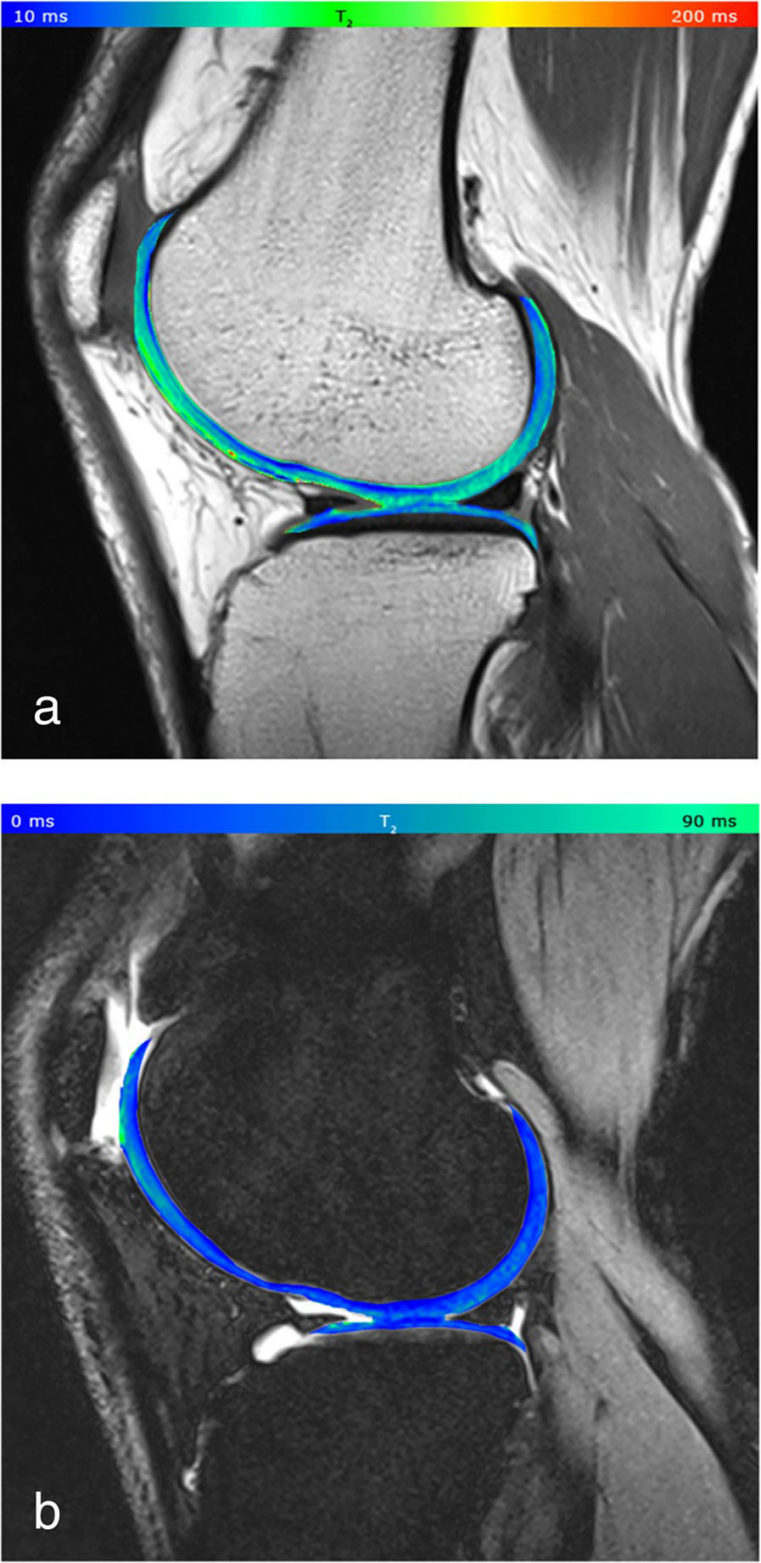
a Cartilage T2 map acquired with a Carr-Purcell-Meiboom-Gill (CPMG) multi-echo spin-echo (MESE) sequence, pseudo-colour-coded and overlaid on a morphological T2-weighted image from a 32-year-old healthy volunteer (echo time 11.9 ms). b Cartilage T2 map acquired with a three-dimensional triple echo steady state, pseudo-colour-coded and overlaid on the second acquired image (F_0_, the lowest order steady state free precession—free induction decay mode acquired in the repetition time). The typical zonal stratification of cartilage is observable with both techniques. However, the CPMG sequence provides apparently higher dynamic contrast of T2 values with better depiction of cartilaginous layers. Reprinted with permission from: Juras et al., Eur Radiol 2016 (reference [21])
